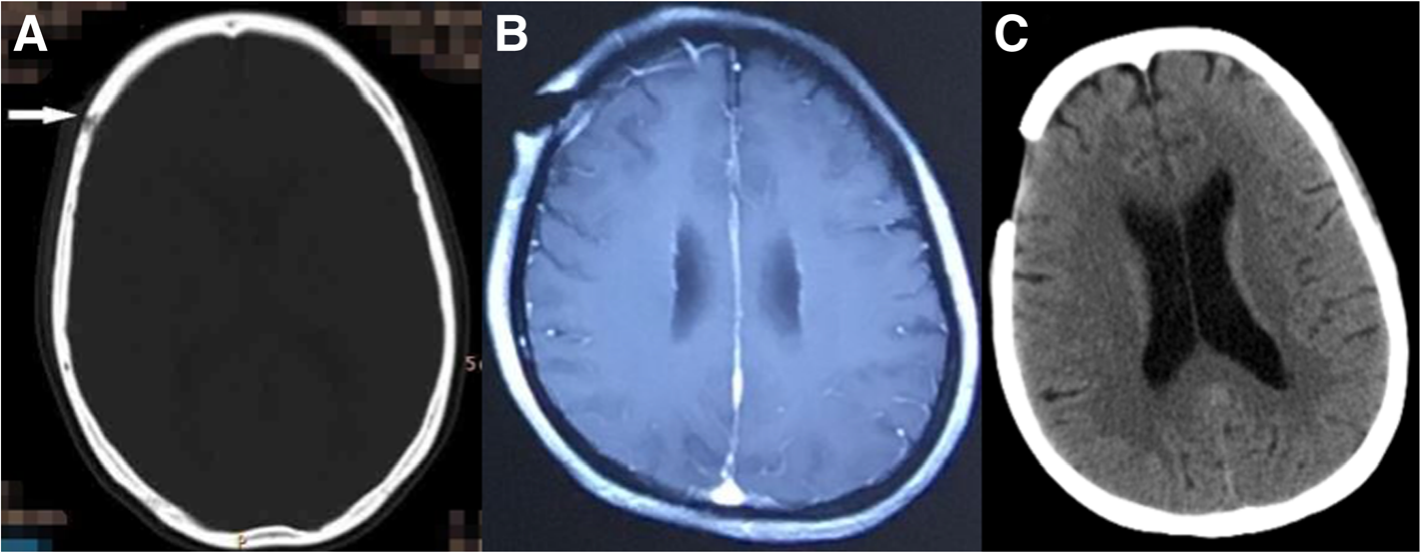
a-c Are computed tomography sequences of the head. a was taken in August 2017, which showed the skull damaged (arrow). b was taken in September 2017, which showed bone loss and destruction at the corresponding place. c was taken in February 2018, showing a postoperative change of the right frontal
